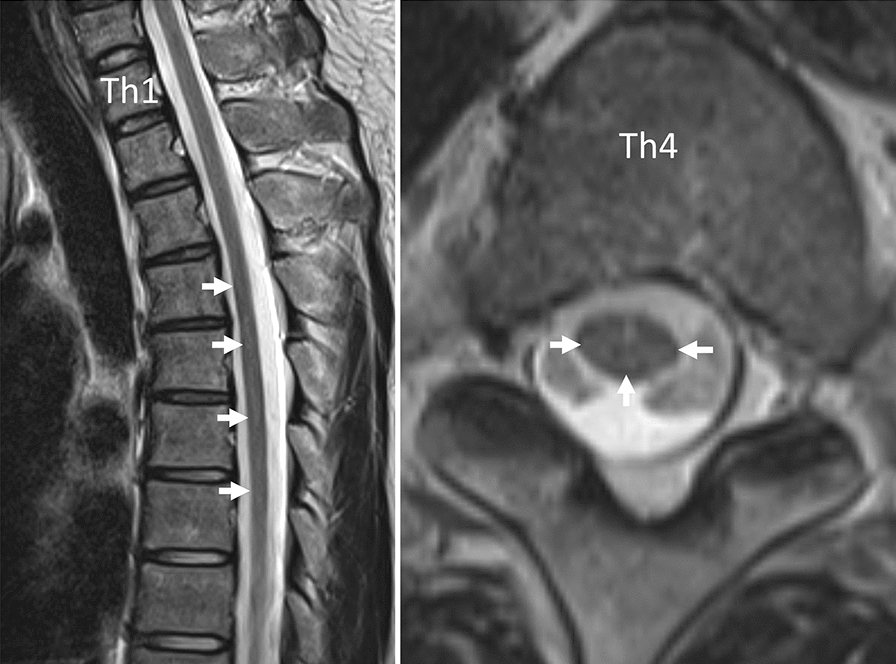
Spine MRI on admission. The sagittal T2-weighed MR image of the spine shows focal demyelinating lesions of the spinal cord from the 4th through the 7th vertebral body (arrowhead), and diffuse spinal atrophy
